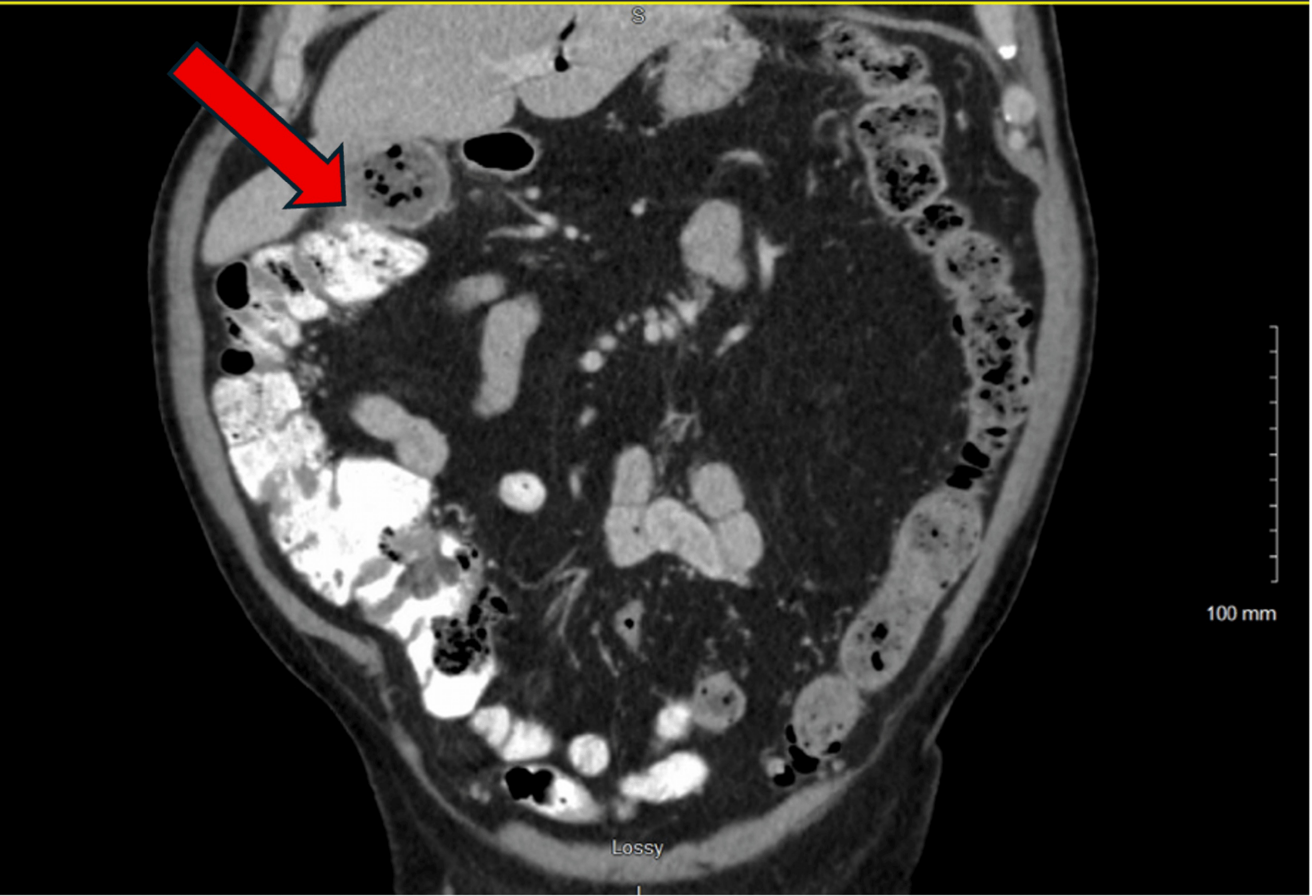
Coronal computed tomography image with intravenous contrast demonstrating a cholecystocolonic fistula in the region of the hepatic flexure.
